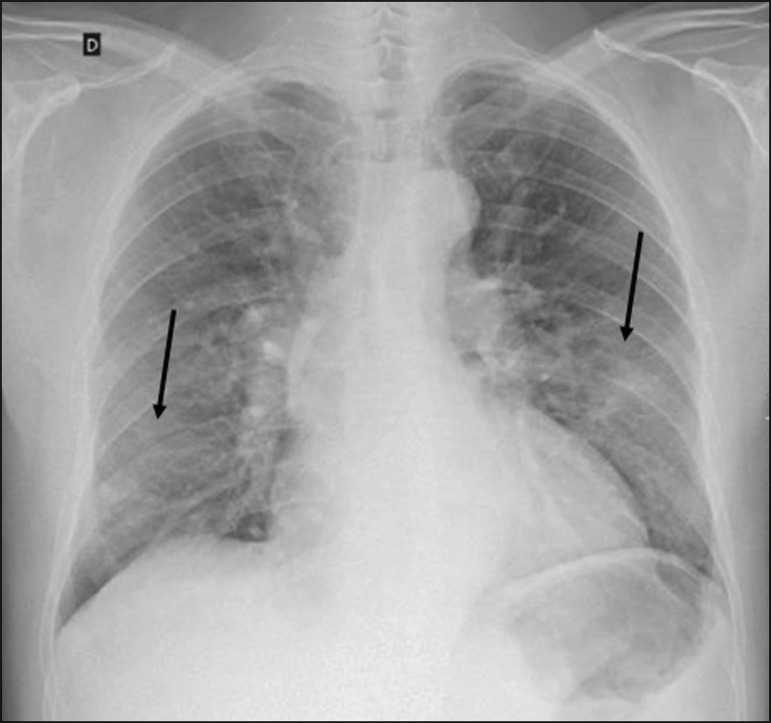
Chest X-ray of a 69-year-old male patient with a 2-day history of cough, adynamia, and fever who tested positive for SARS-CoV-2 on RT-PCR, showing low-attenuation pulmonary opacities (arrows) in the lung bases, more pronounced on the left.
